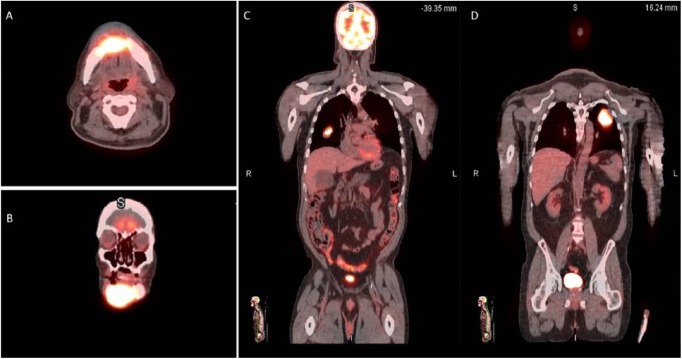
Axial (A) and coronal (B) positron emission tomography-computed tomography (PET-CT) demonstrating a metabolically active lesion in the anterior mandible with body PET-CT (C, D) demonstrating hypermetabolic lesions in the lung concerning for metastasis. Note the absence of hypermetabolic lesions in the pelvis.
